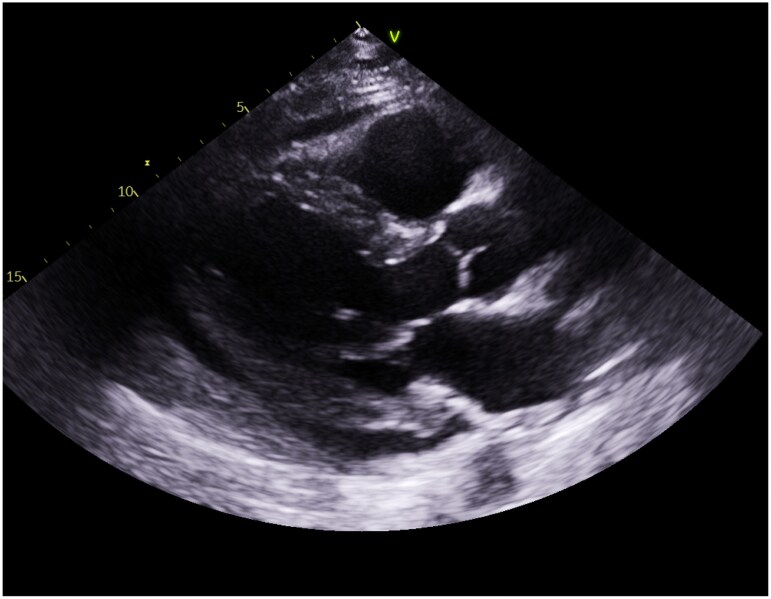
Echocardiogram showing improvement post-initial medical management.
